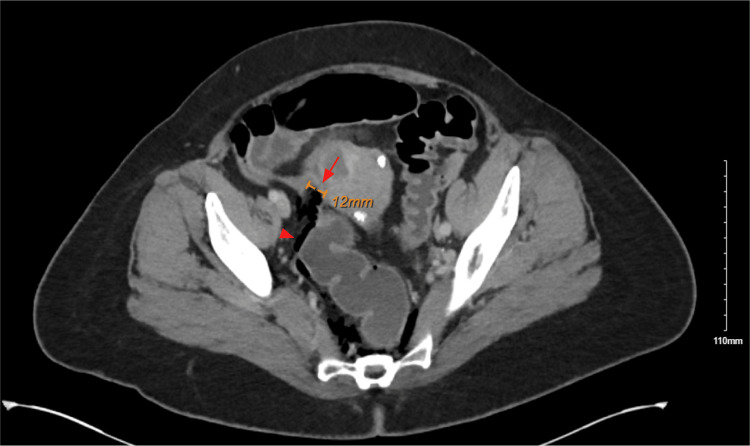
Preoperative CT of the abdomen/pelvis demonstrating sigmoid perforation and free intraperitoneal air
